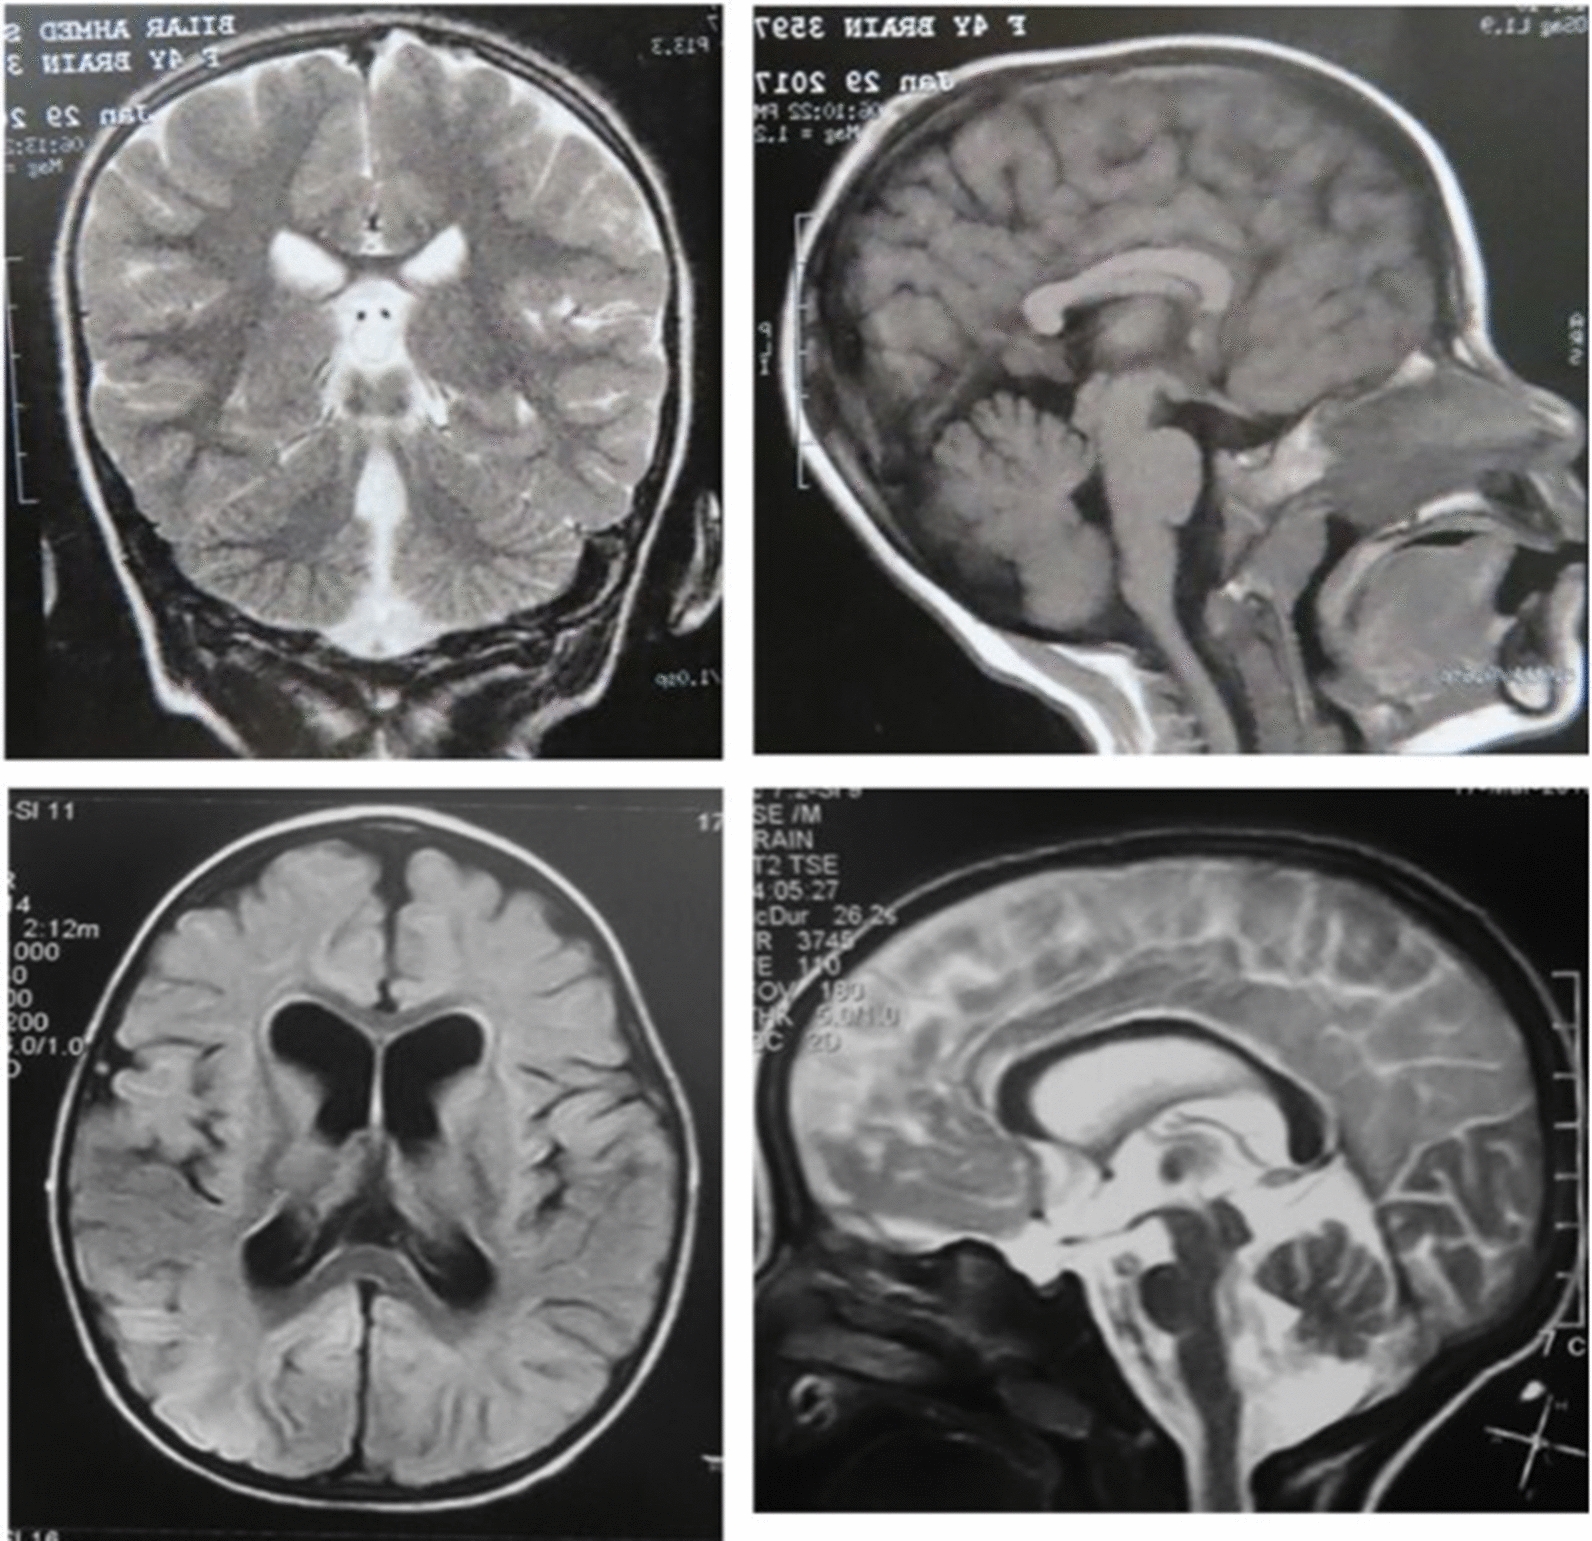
MRI images of the studied CDG cases. Top images: The SLC35A2-CDG patient showing a short malformed corpus callosum and an inferior vermis hypoplasia. Bottom images: The SRD5A3-CDG patient showing a mild prominent cortical sulci frontally dilated lateral ventricles and a significant reduction of vermian size
